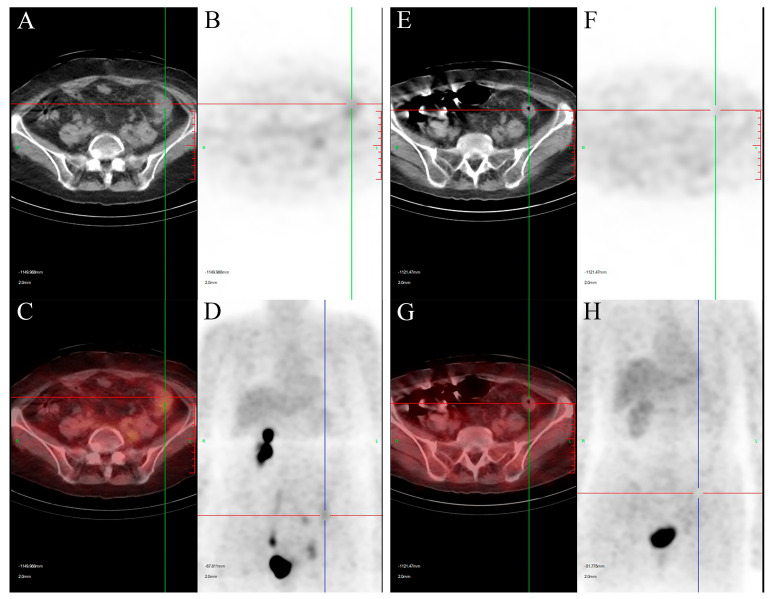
PET/CT evaluation of the descending colon lesion. Left panels (pre-treatment): (A) Axial CT shows localized wall thickening of the descending colon with blurred surrounding fat planes. (B) Corresponding PET image shows focal abnormal radiotracer uptake in the colonic lesion. (C) Fused image confirms colocalization of the hypermetabolic area with the anatomical abnormality. (D) Whole-body maximum intensity projection (MIP) image highlights the hypermetabolic descending colon lesion. Right panels (post-treatment): (E) Axial CT image shows significant improvement in pericolonic infiltration. (F) Axial PET and (H) MIP images demonstrate markedly reduced metabolic activity in the colonic lesion. (G) Fused image confirms reduced metabolic activity and lesion size.
